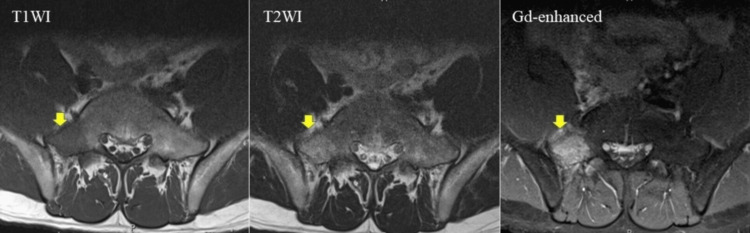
MRI before chemotherapy MRI showed a T1 low signal, T2 high signal and Gd contrast effect on the right side of the sacrum.
